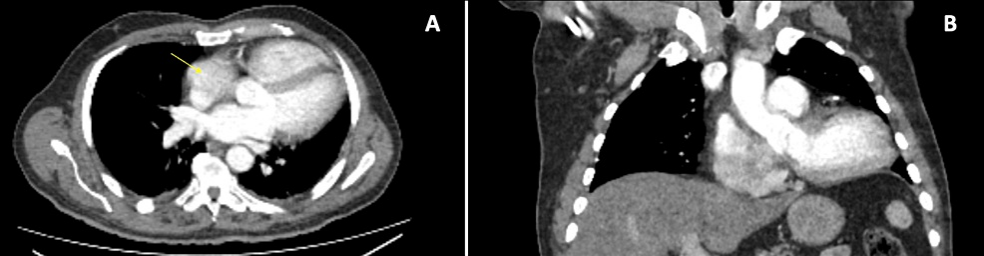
Preoperative axial (A) and coronal (B) CT images of the thorax. The position of the heart is more leftward with the right atrium in the midline.
